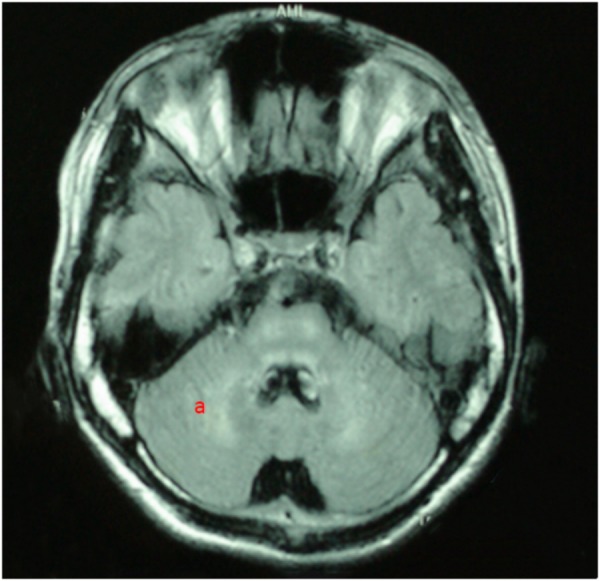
T2WI FLAIR image in a 19-year-old male. axial images show hyperintensity in cerebellum (a).
